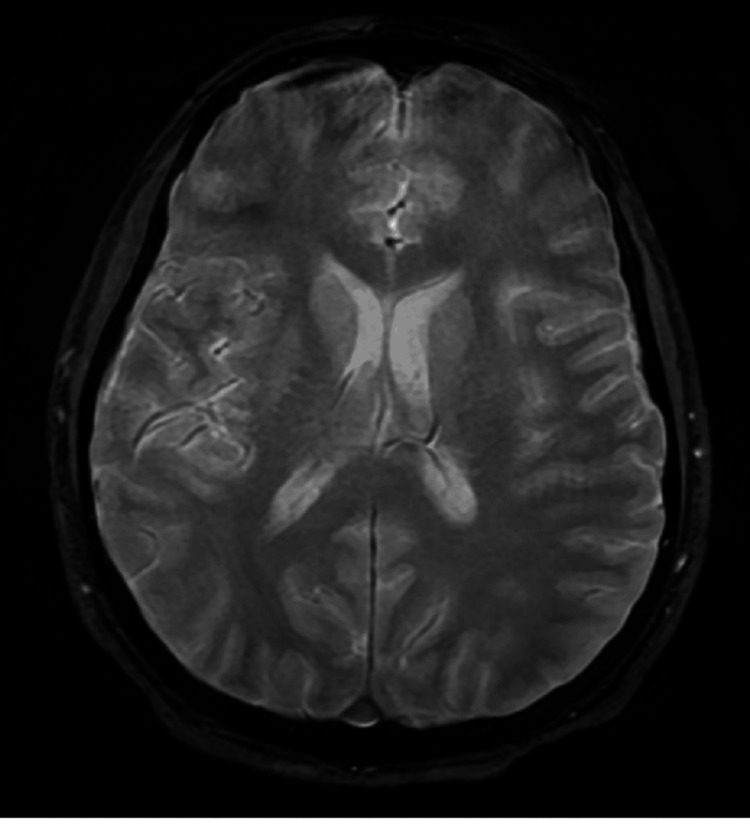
Axial T2-weighted brain MRI This axial T2-weighted image demonstrates structurally normal brain anatomy with no evidence of acute infarction, hemorrhage, or encephalitic changes. The ventricles appear normal in size and shape, with no signs of hydrocephalus or midline shift. Despite the absence of overt abnormalities, diagnostic interpretation was hindered by motion artifacts, likely due to the patient's reduced level of consciousness and need for sedation, limiting the sensitivity of the scan in detecting subtle parenchymal changes. In the context of the patient's clinical presentation, the normal MRI underscores the challenge of radiological diagnosis in viral encephalopathy and highlights the importance of EEG and CSF analysis in reaching a clinical diagnosis. MRI: magnetic resonance imaging; EEG: electroencephalogram; CSF: cerebrospinal fluid
